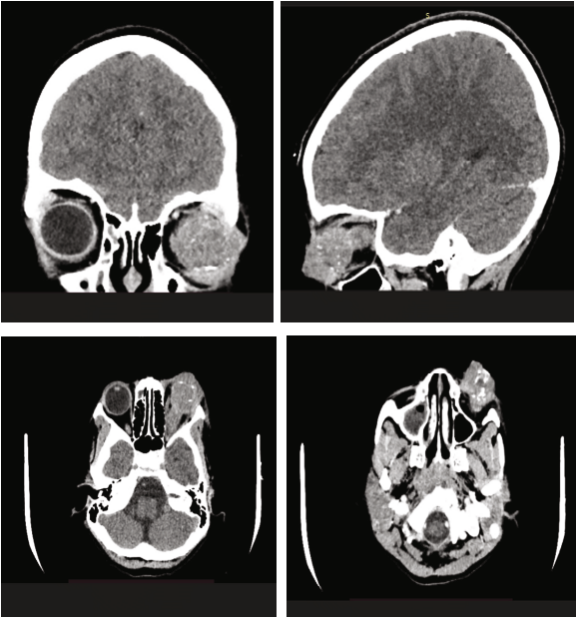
Computed tomography (CT) scan of the orbit and brain. A lobulated hyperdense mass involving the left globe and optic nerve with loss of globe contour, optic nerve thickening, and multifocal intraocular calcifications. The right orbit and extraocular muscles appear normal.
